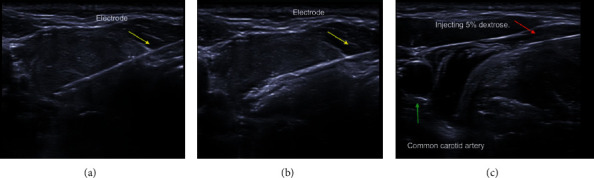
A 37-year-old woman presented with neck bulging. (a) Axial sonographic image shows the trans-isthmic approach of 5 mm size active tip of the electrode (yellow arrow). (b) Axial sonographic image shows the moving-shot technique (yellow arrow). After ablation of the peripheral imaginary unit, the electrode is moved to the central portion and ablation is performed. (c) The hydrodissection technique was applied to preventing important structures by injecting slowly 10 ml 5% dextrose (red arrow).
